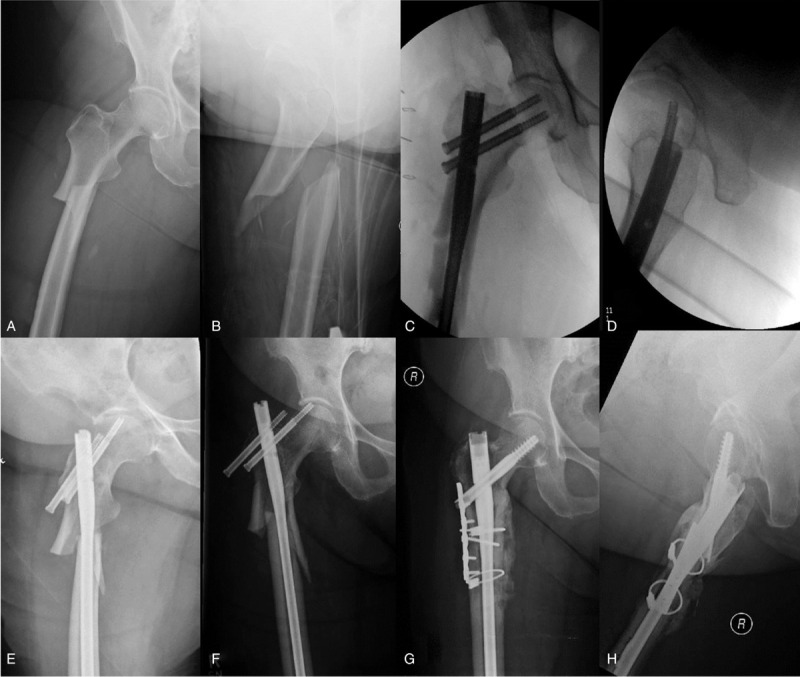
Atypical femur fracture nonunion. Radiographs of a 78-year-old female after a low-energy fall demonstrating an atypical femur fracture in the subtrochanteric region (A and B). She had an 8-year history of alendronate use for osteoporosis, and a 3-week history of prodromal right thigh pain prior to fracture. She underwent closed reduction internal fixation with a cephalomedullary nail (C and D). Three-month follow-up radiographs demonstrate progressive varus collapse and hardware failure (E and F). Her ESR was 55 mm/h, and CRP was 13 mg/L. She was taken for revision fixation, including complete hardware removal, irrigation, and debridement with multiple deep tissue cultures. Revision nailing was performed following resection of a small amount of lateral bone and the use of a lateral unicortical plate to correct the previous varus malalignment. Cerclage cables were used to reduce and provide fixation of the large calcar fragment (G and H). Local intrawound vancomycin powder and bone morphogenetic protein (BMP, Infuse, Medtronic) were used to prevent/treat infection and augment biology. Two of the 5 deep intraoperative cultures were found to be positive for *staphylococcus epidermidis*. She received 6 weeks of tailored intravenous antibiotic therapy postoperatively. At 6 months postoperatively, radiographs show solid union and the patient was clinically free of infection.
